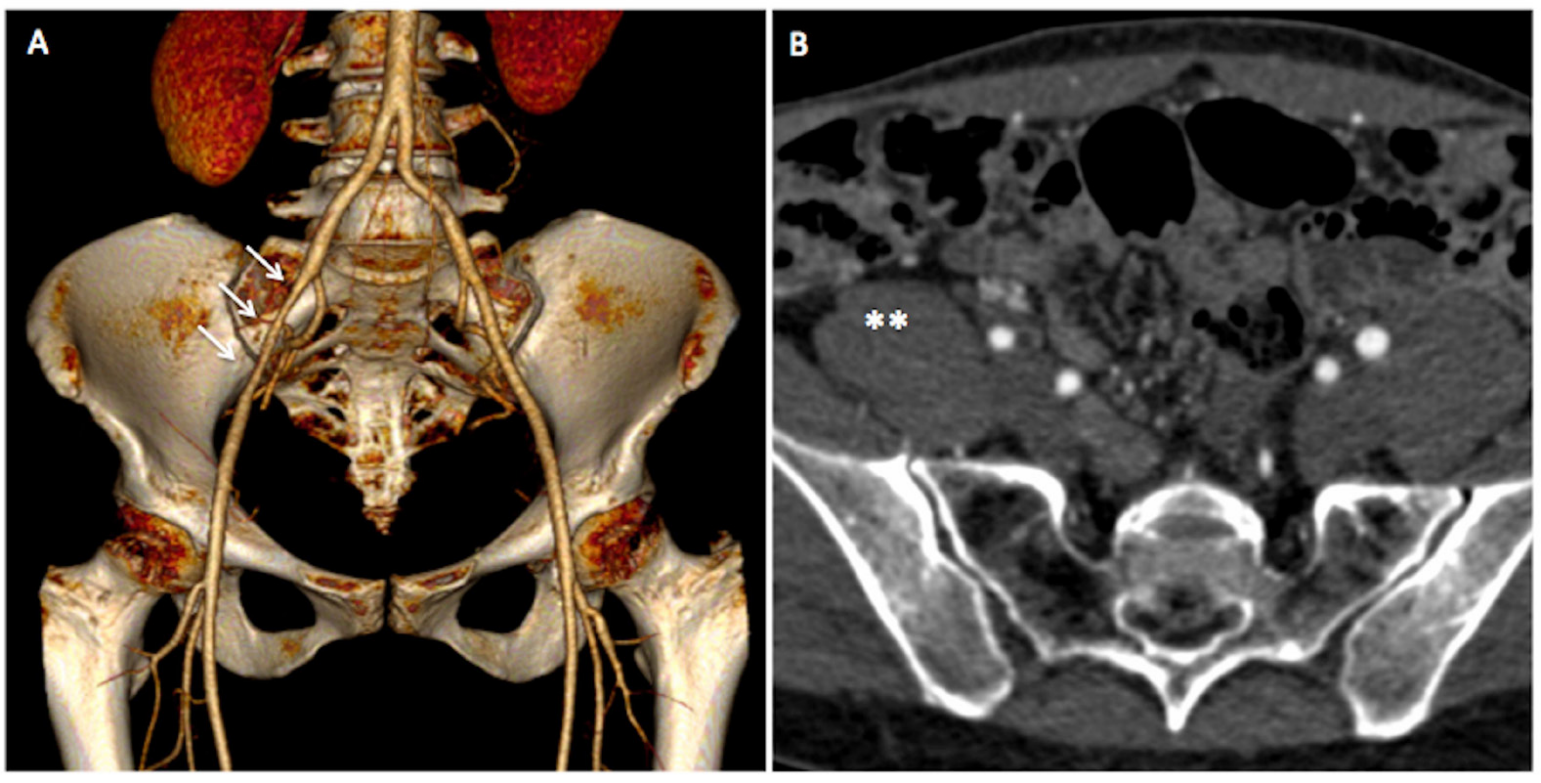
(A) Three-dimensional CT angiographic reconstruction, demonstrating narrowing of the right external iliac artery (arrows); (B) Axial CT image showing right external iliac artery stenosis with a hypertrophic psoas muscle ().
